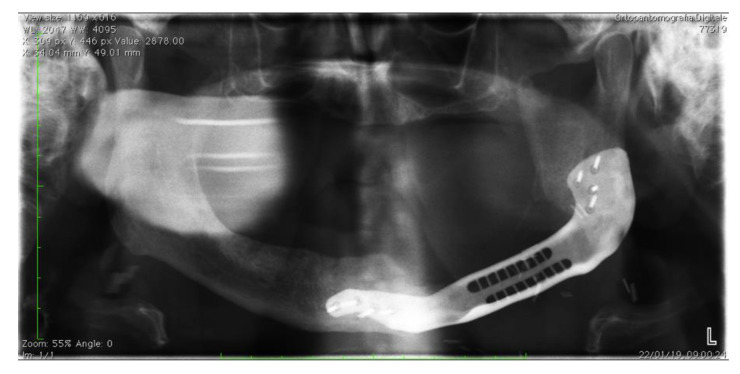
Postoperative orthopantomography in a patient treated for left-body biphosphonate-related osteonecrosis of the mandible (BRONJ).
